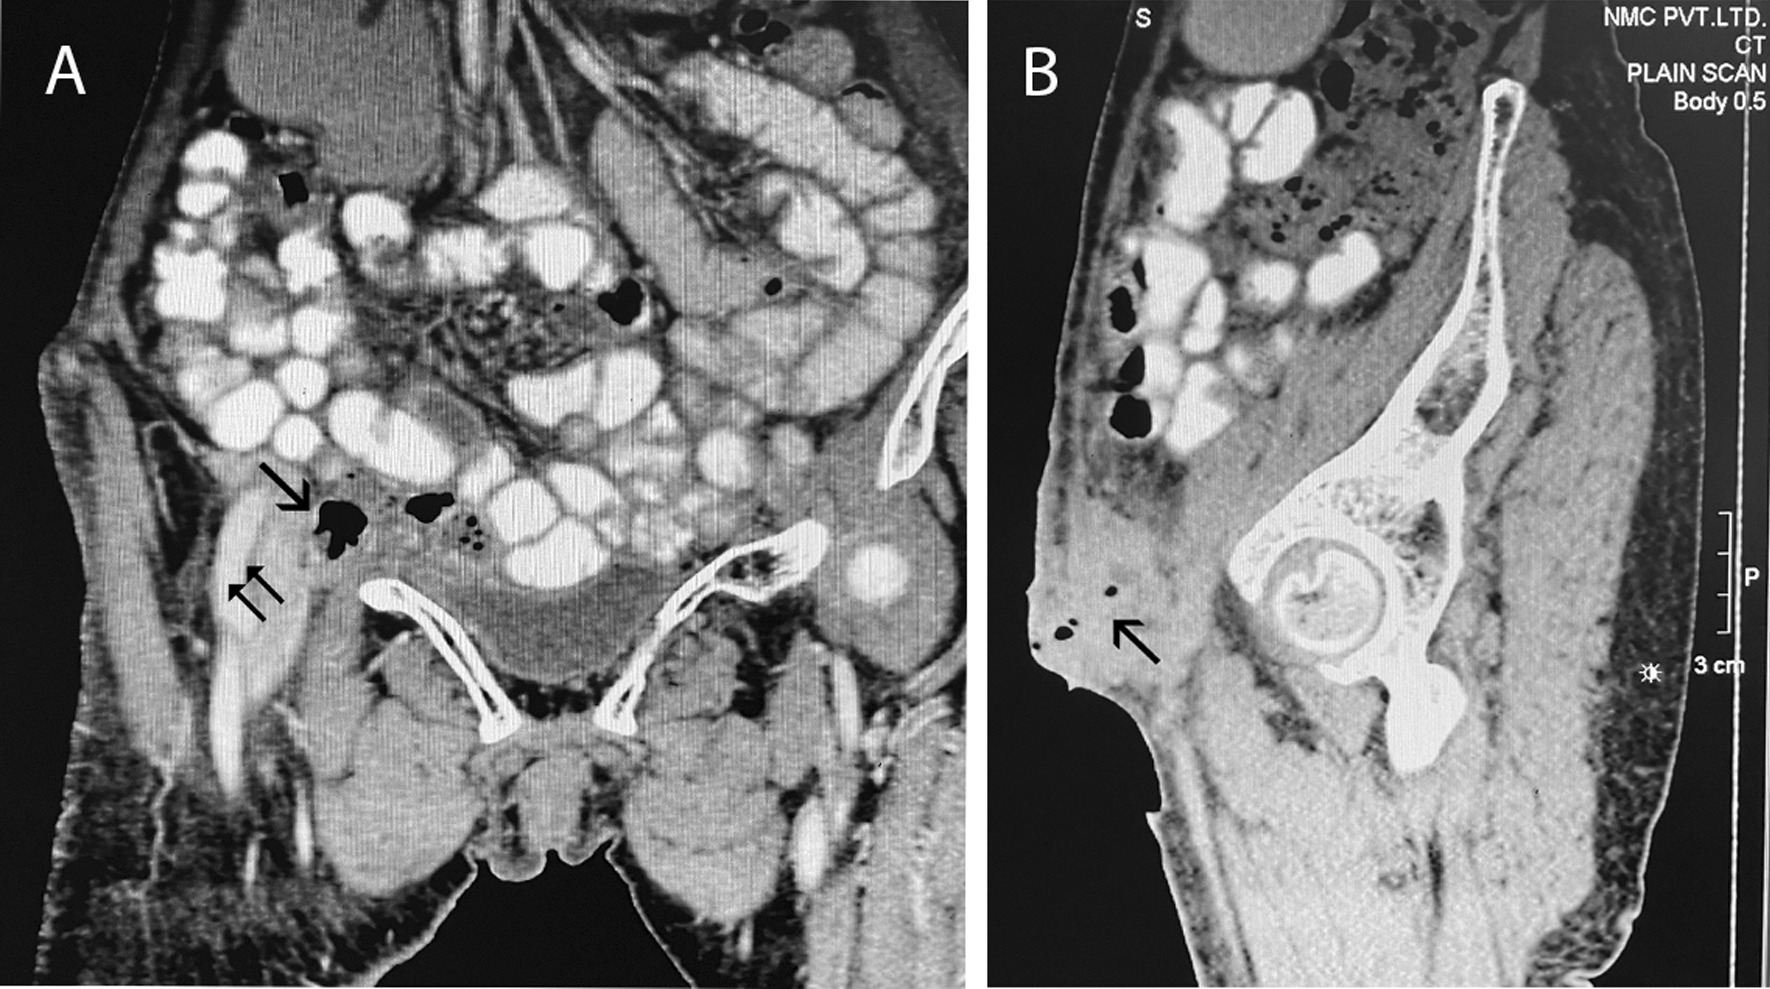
CT scan findings showing location of fistulation and femoral hernia
